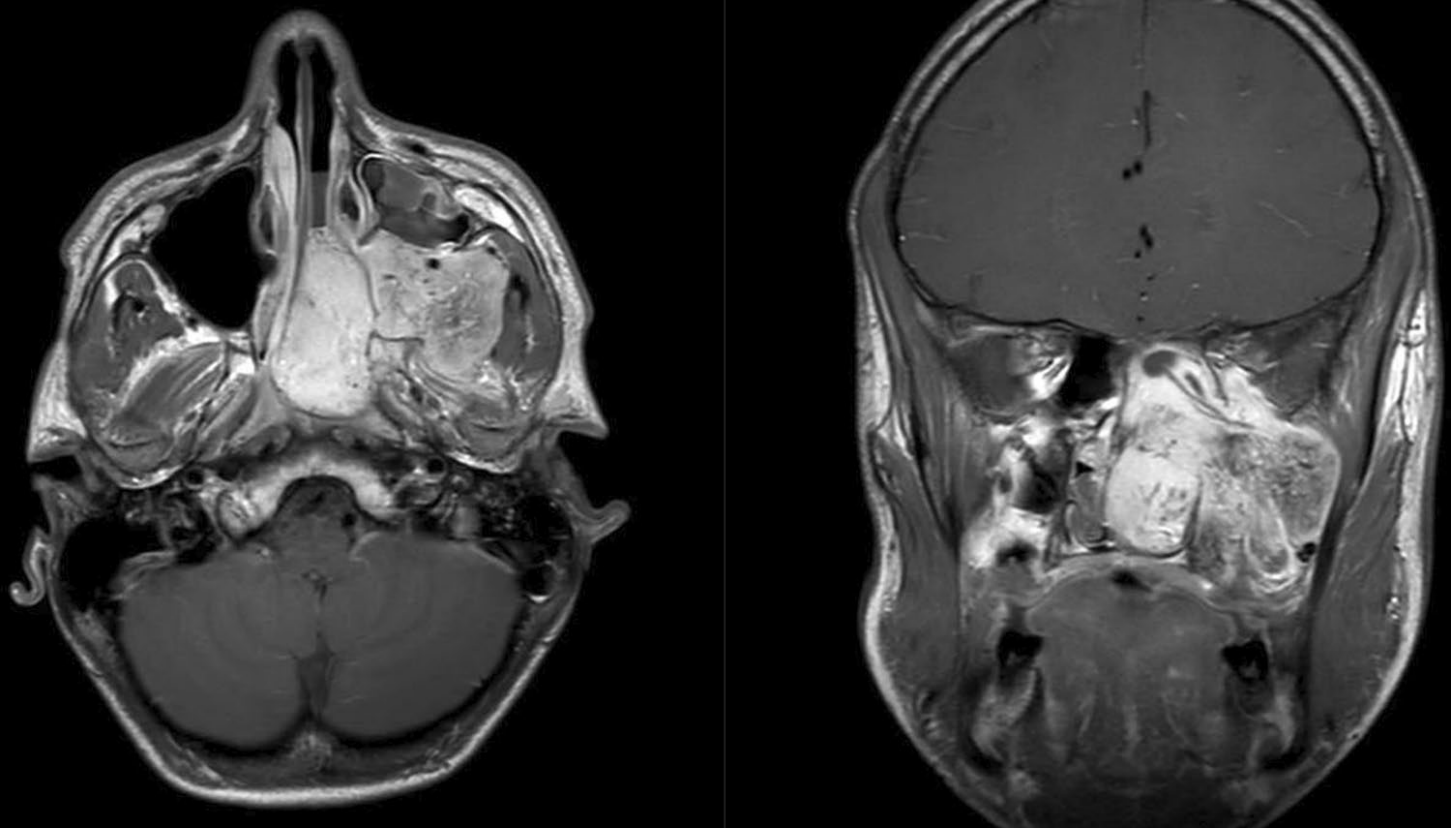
MRI of the juvenile nasopharyngeal fibroma in the left nasal cavity, nasopharynx and pterygopalatine fossa
